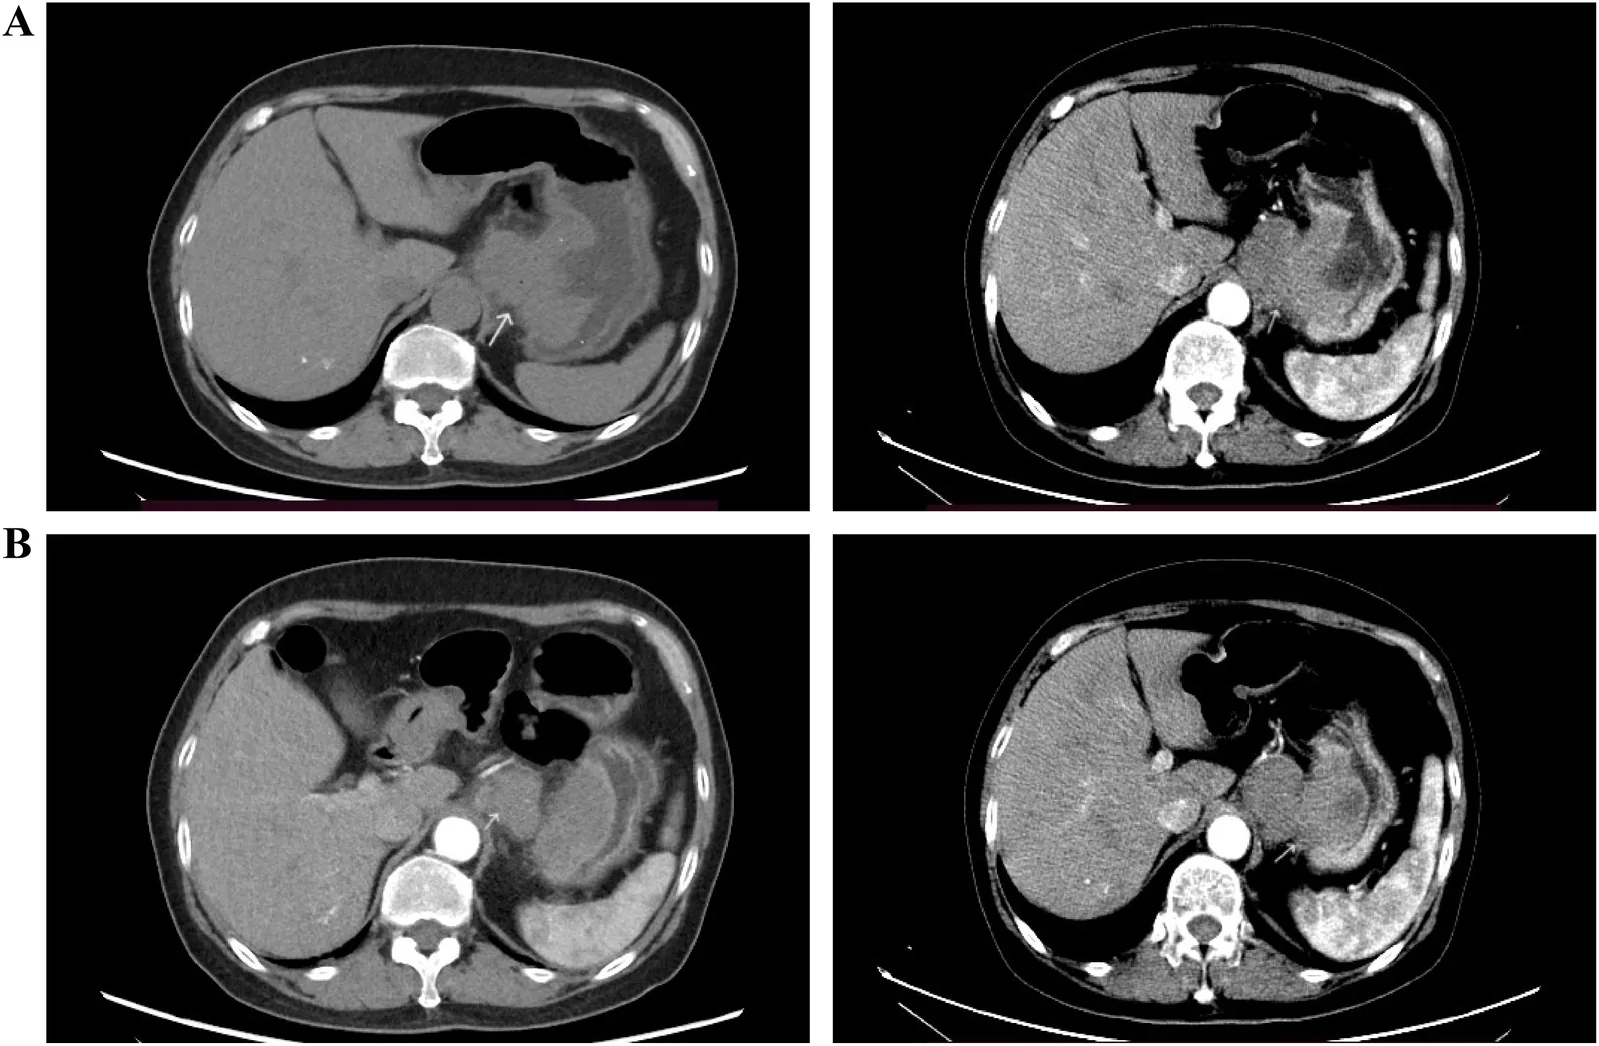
Computed tomography (CT) showed: (A) 2022.12 (right) and 2023.01 (left), and the wall thickness of the lower esophagus, cardia, and stomach was about 5.42cm, which was significantly larger than that of the anterior (4.32cm). (B) 2022.12 (right) and 2023.01 (left), and there were multiple enlarged lymph nodes around the stomach, with a short diameter of about 1.5cm, larger than that of the anterior (1.2cm).
